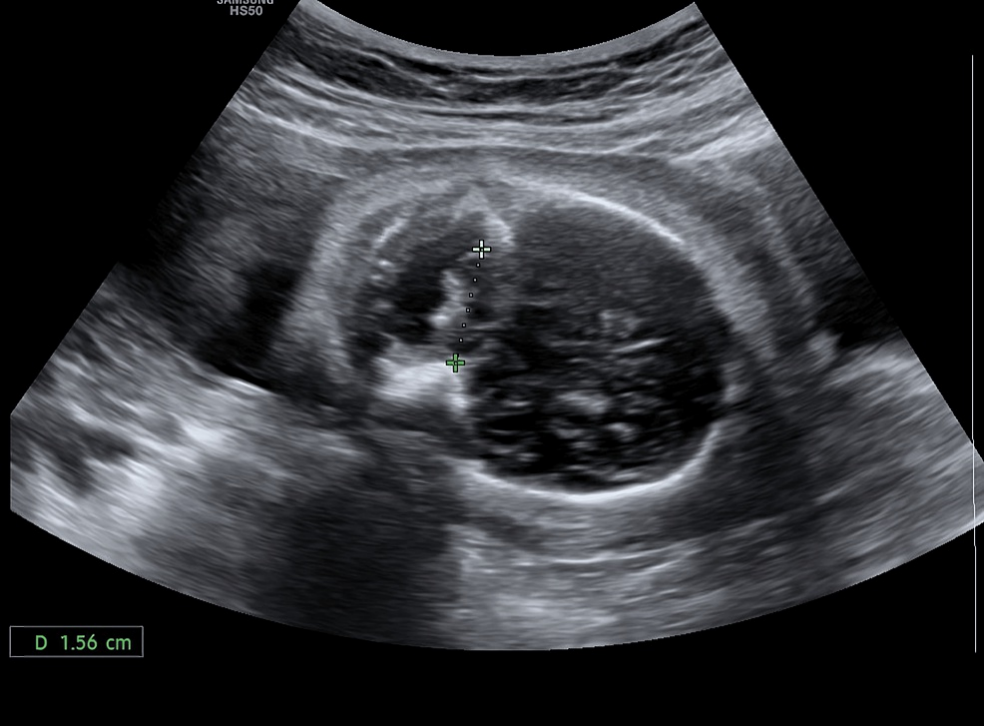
Fetal neuro-sonogram Trans-cerebellar diameter measuring 1.56 cm at 25 weeks gestation.
